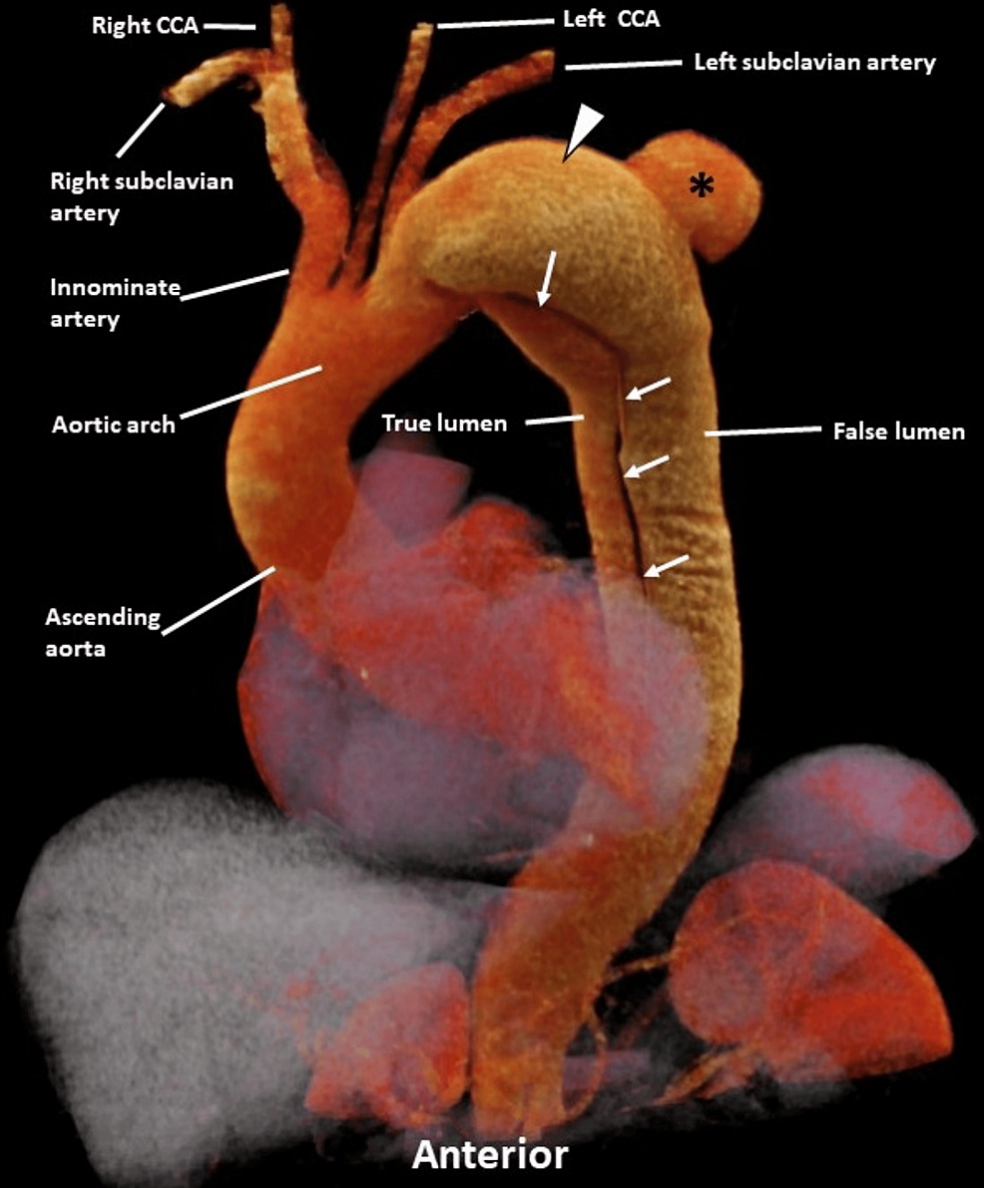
A three-dimensional cinematic volume-rendered image (SIEMENS Healthineers, Erlangen, Germany) of CT angiography of the chest following the injection of 80 mL of intravenous contrast iopamidol 76% (Isovue 370, Bracco) into the right upper extremity reveals a type B aortic dissection. The dissection flap extends distally from the origin of the left subclavian artery (solid white arrows). There is also a localized saccular outpouching indicating contained rupture (black asterisk) from the posterior false lumen of the proximal descending thoracic aortic dissection, accompanied by aneurysmal dilation (white arrowhead). CCA: common carotid artery
